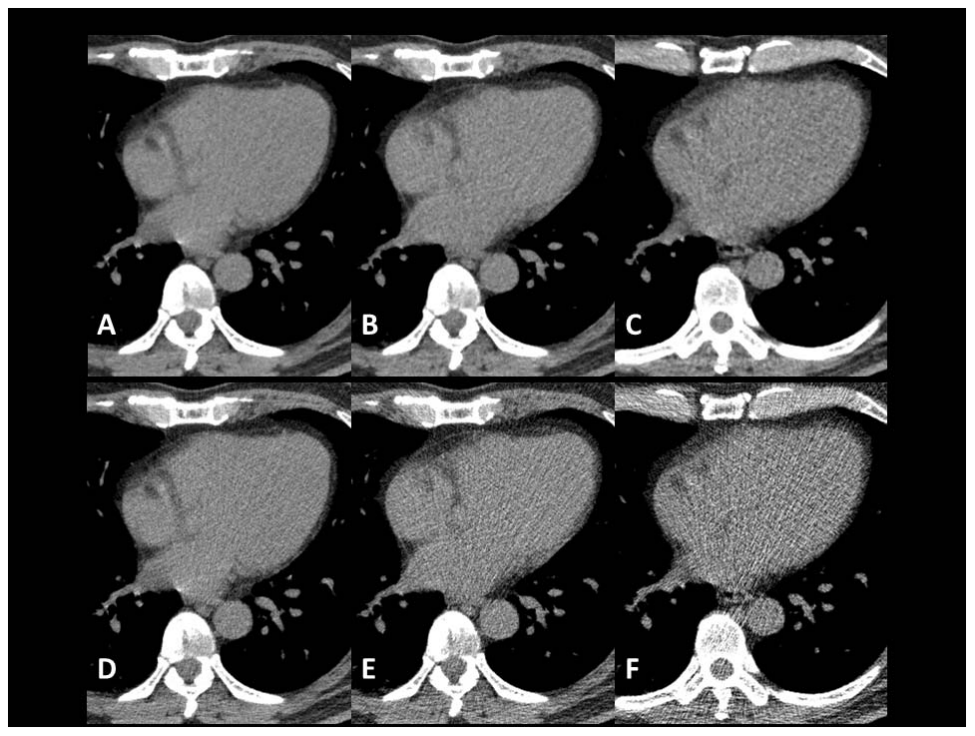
Axial plain chest CT images with a mediastinal setting to assess streak artifacts (55-year-old male weighing 64 kg). A , at 240 mA with AIDR3D; B, at 120 mA with AIDR3D; C, at 60 mA with AIDR3D; D, at 240 mA without AIDR3D; E, at 120 mA without AIDR3D; F, at 60 mA without AIDR3D. Many radial streaks from the spine were apparent in the heart, particularly on the image without AIDR3D at 60 mA (F). These streaks were greatly reduced using AIDR3D (C, at 60 mA).
